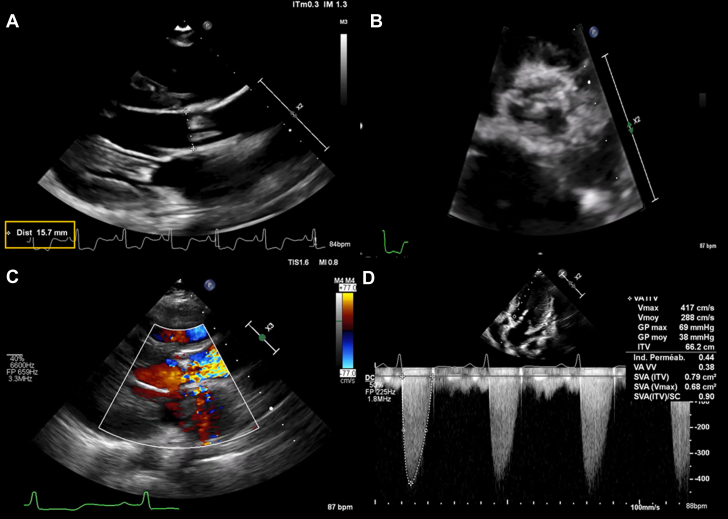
Transthoracic Echocardiography (A) Small aortic annulus (parasternal long-axis view). (B) Severe calcification of the aortic valve leaflets (parasternal short-axis view). (C) High-velocity jet through the aortic valve with a mosaic pattern indicating turbulent flow (color Doppler, parasternal long-axis view). (D) Doppler profile consistent with severe aortic stenosis (continuous-wave Doppler, apical 5-chamber view).
